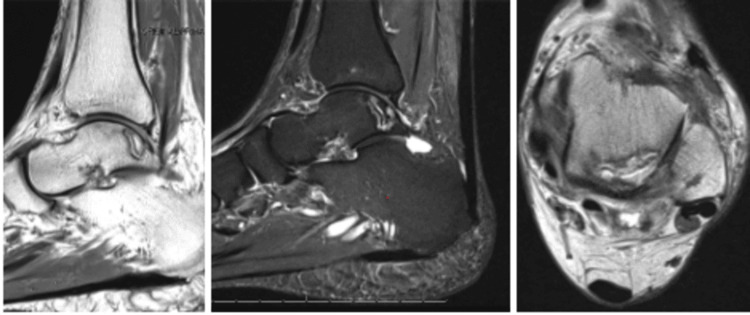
MRI from July 12, 2023, demonstrates resolution of tibial AVN, healing of the talus fracture with persistent AVN posterior lateral talar dome. AVN: avascular necrosis
